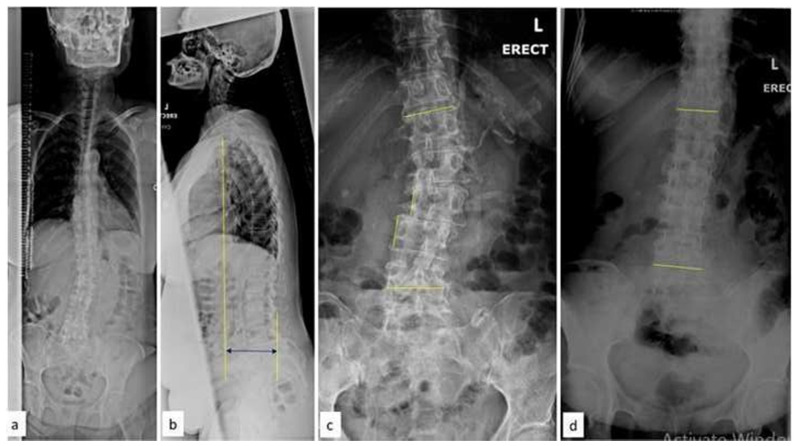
(a–b) Whole Spine AP and lateral radiographs with degenerative lumbar scoliosis. Note the CSVL anterior to the postero-superior aspect of S1 and the femoral heads (c) AP radiograph showing the magnitude of coronal deformity and lateral listhesis at L3–L4 level (d) AP radiograph of the lumbar spine after the first stage OLIF surgery. Note the significant correction of the deformity in coronal plane.
